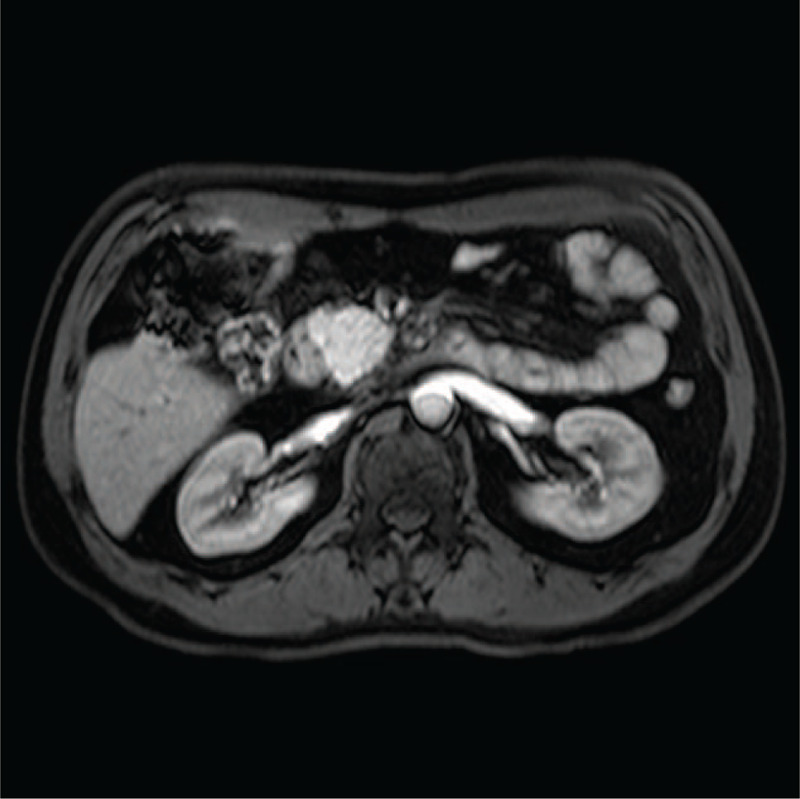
Contrast-enhanced magnetic resonance imaging showing thickening and luminal narrowing of the descending part of the duodenum.
